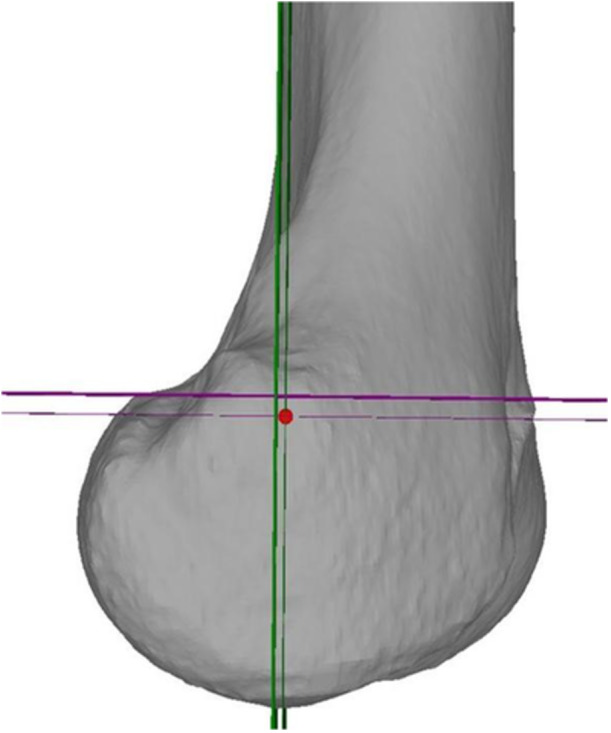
Determination of the radiographic Schoettle's point (red) according to the posterior femoral cortical line (green, thick) and 1.3 mm anterior to the posterior femoral cortical line (green, thin) as well as the perpendicular epicondylar line (purple, thick) and 2.5 mm distal to the perpendicular epicondylar line (purple, thin).
